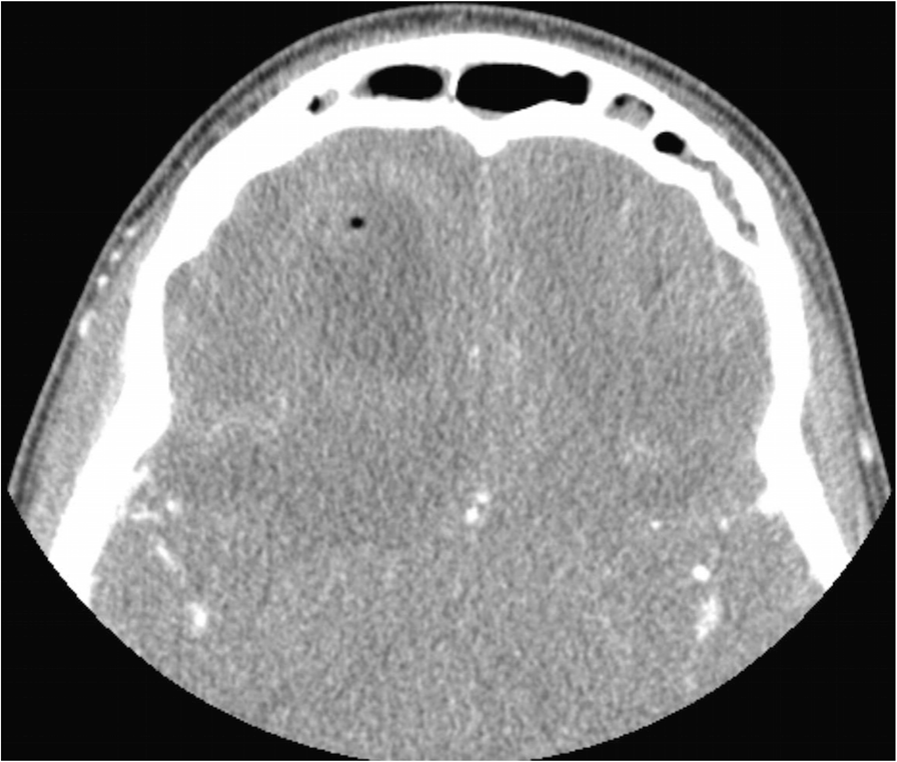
CT maxillofacial, axial view, demonstrating the finding of bifrontal rim-enhancing fluid collections
